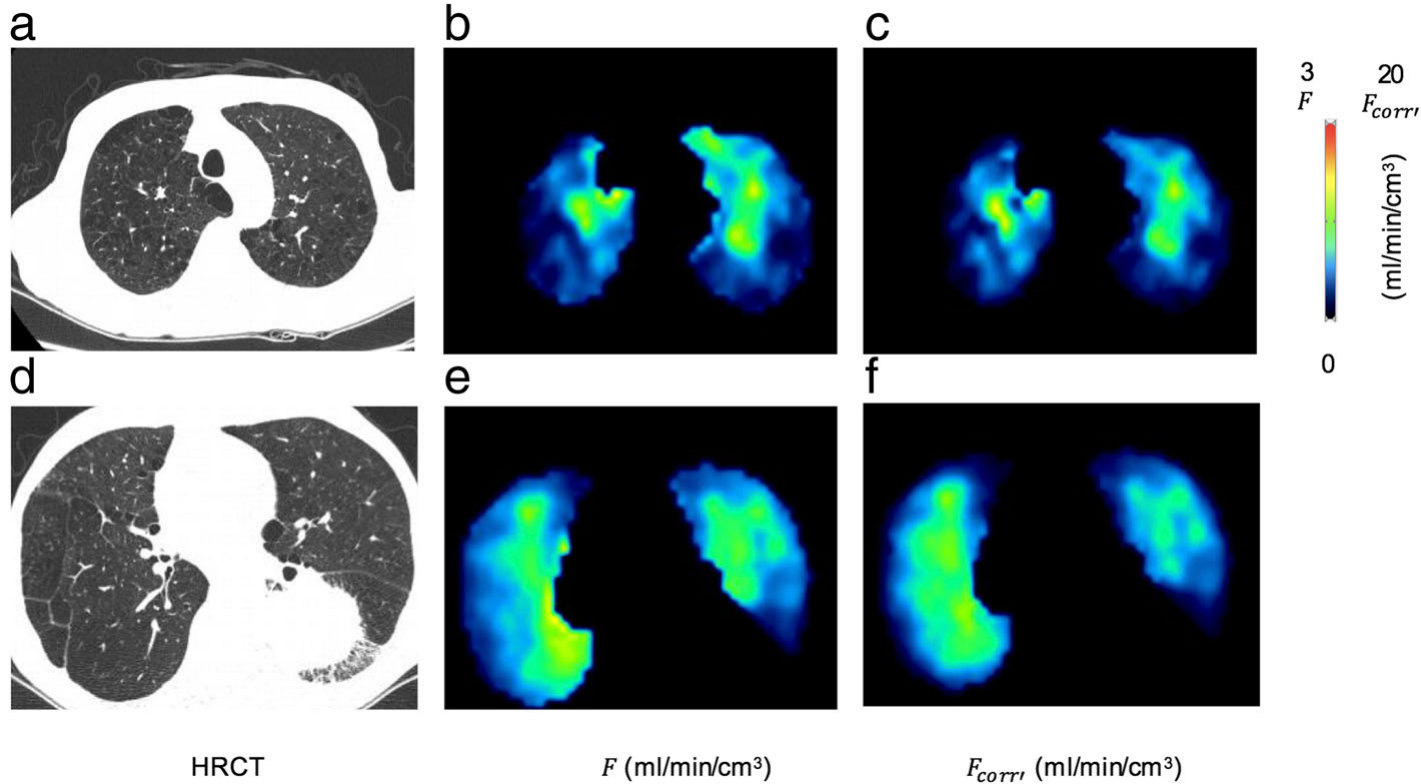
HRCT image (a), PBF image without TF correction (b), PBF image with TF and blood volume correction (c) of apical area and those of basal area (d-f) in patient 9, who had left lower lobe lung cancer and emphysematous changes
